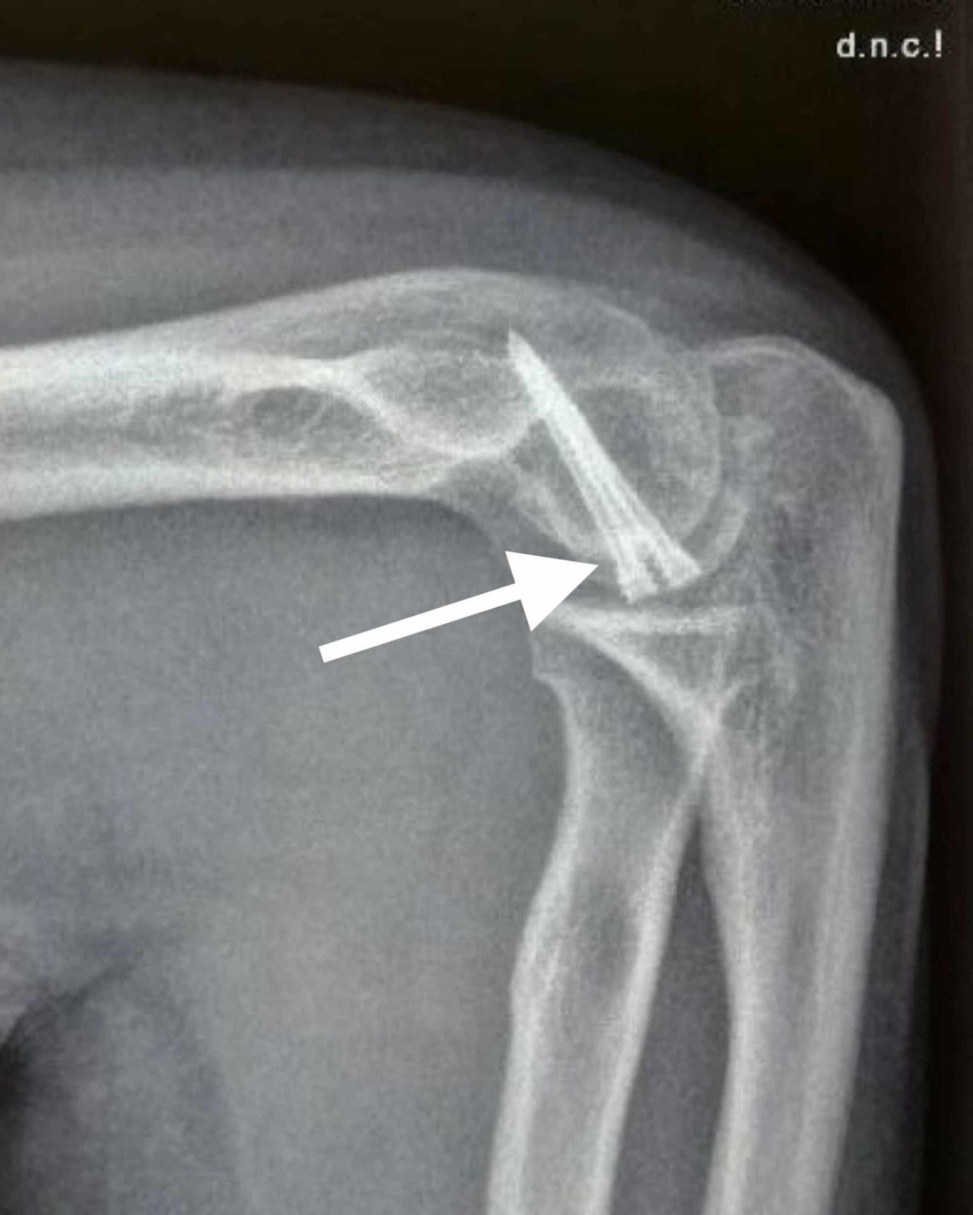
Postoperative X-ray radiograph lateral view showing a fracture of the capitellum managed with Herbert screw fixation
